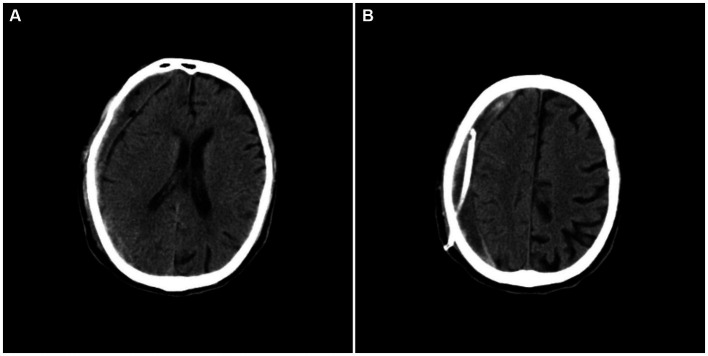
Postoperative craniocerebral CT revealing that the subdural hematoma was smaller in size, the compression of the lateral ventricle was relieved, and the degree of displacement of the midline structure was reduced.
